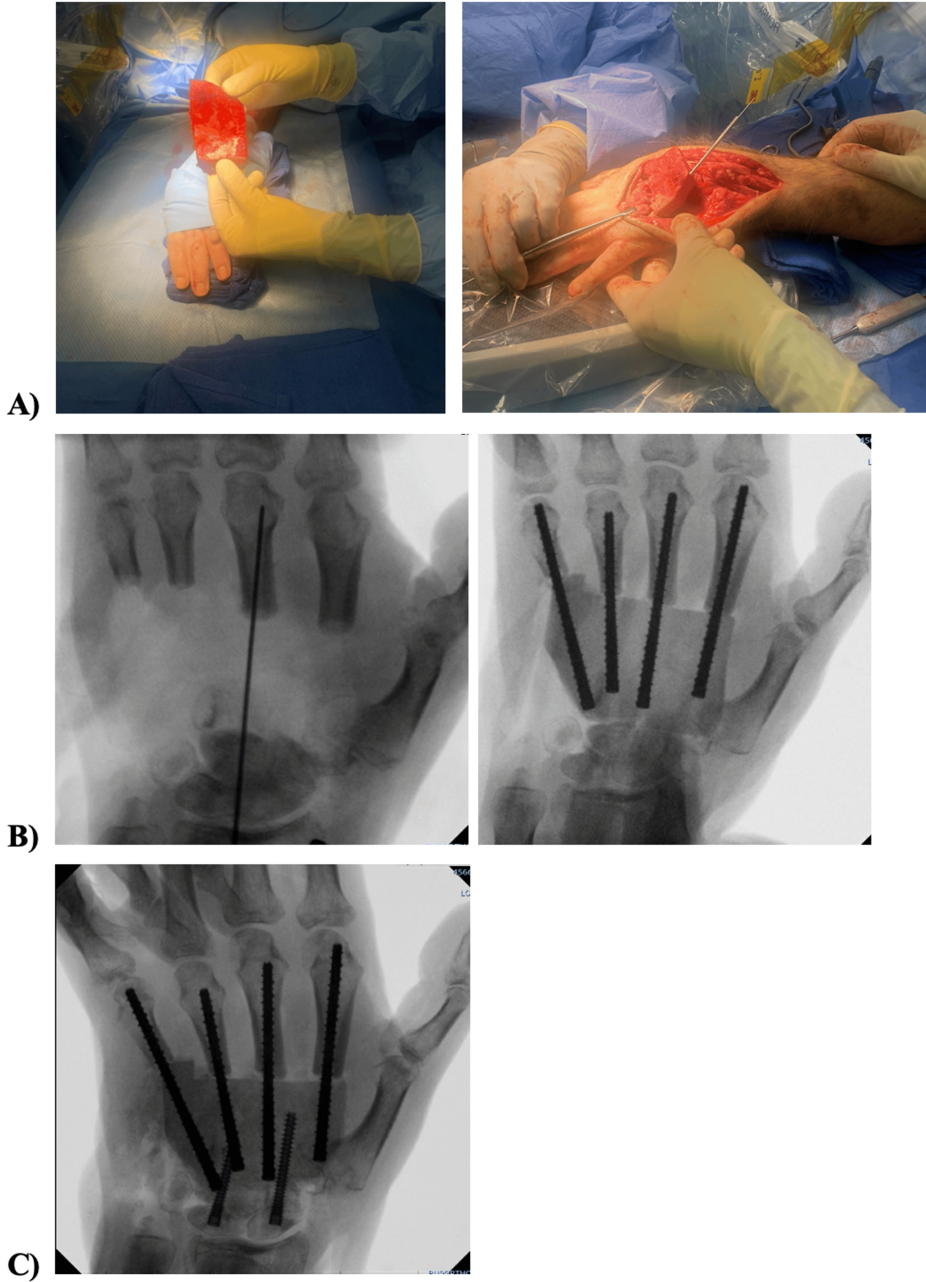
(A) Intraoperative clinical photo of iliac bone graft fixed in place with intramedullary metacarpal screws. (B) Radiographs of iliac bone graft fixed in place with intramedullary metacarpal screws. (C) Intraoperative radiograph of metacarpal intramedullary screws in second through fifth digits and screws from proximal carpal row into the iliac bone graft.
